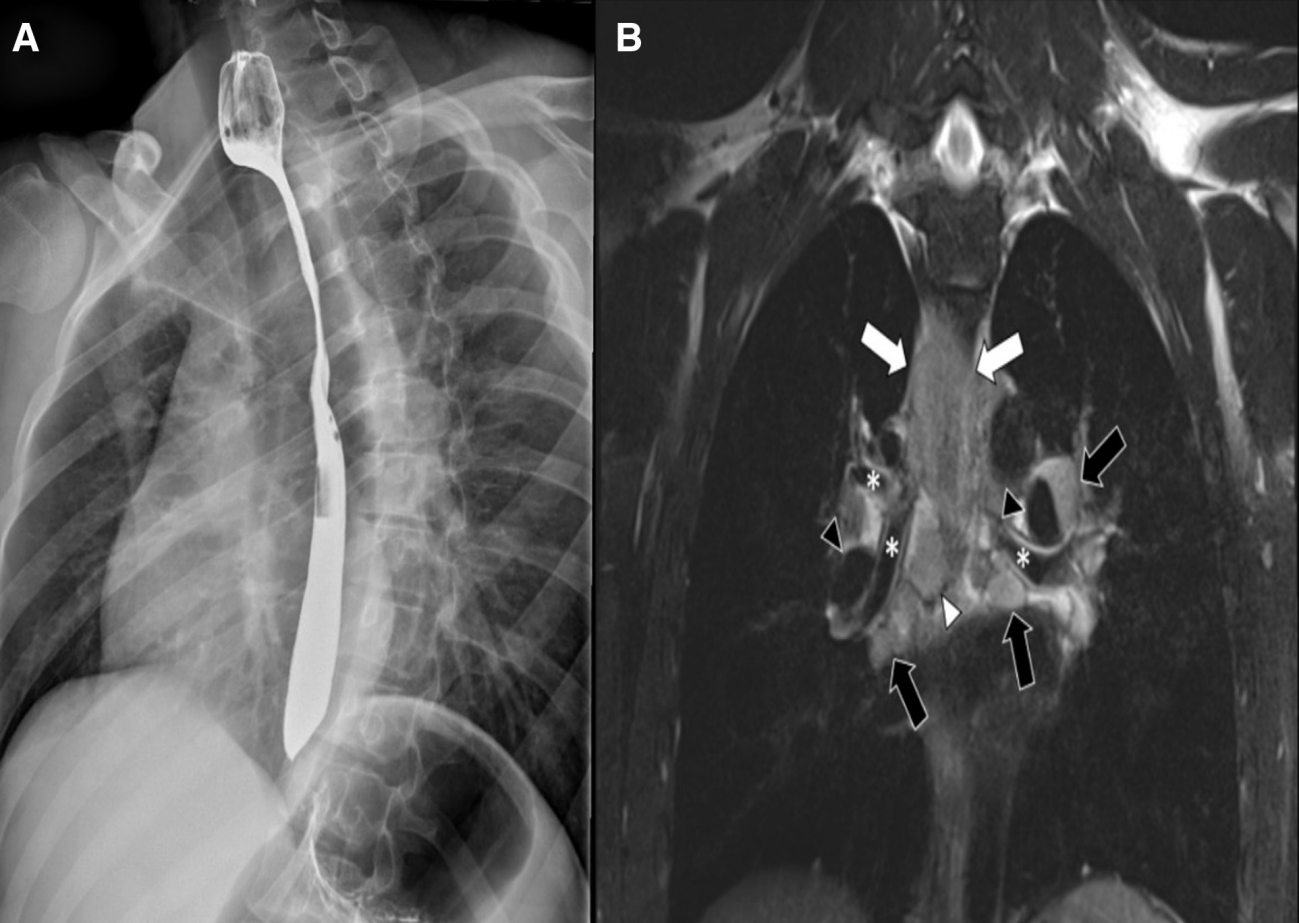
A) Esophagram performed at an outside hospital revealing a 7-cm stricture beginning at the thoracic outlet and extending caudally. B) Coronal MR image (T2-weighted HASTE) again demonstrates the circumferential esophageal wall thickening (white arrows). There are enlarged hilar lymph nodes bilaterally (black arrows) along the bronchi (white asterisks) and pulmonary arteries (black arrowheads) as well as an enlarged subcarinal lymph node (white arrowhead). Given the presence of lymphadenopathy outside the drainage pattern of the esophagus, lymphoma is the leading differential consideration on imaging with primary esophageal malignancy favored to be less likely.
